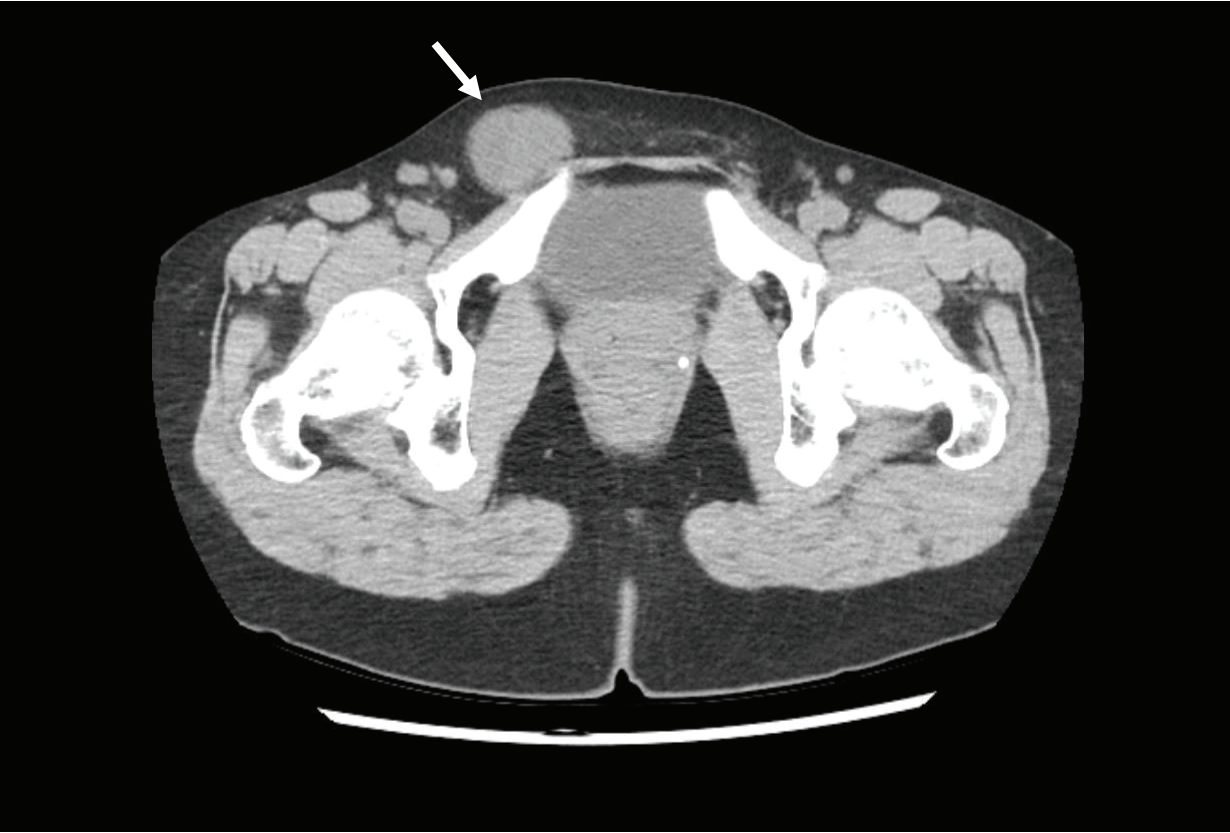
Preoperative CT findings. CT image showing a homogeneous neoplastic lesion measuring 30 × 40 mm along the uterine cord in the right inguinal region.
